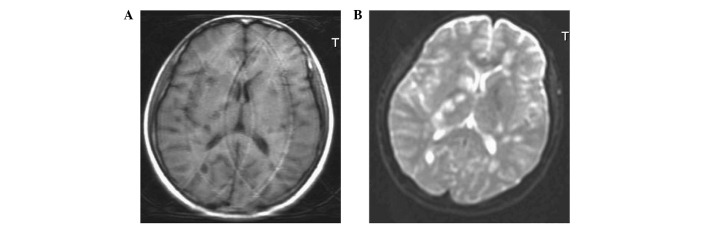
Case 3: (A) Axial T1-weighted imaging (T1WI) demonstrates multiple hypointense foci at the bilateral basal nuclei; and (B) diffusion-weighted imaging (DWI) exhibits hyperintense foci.
